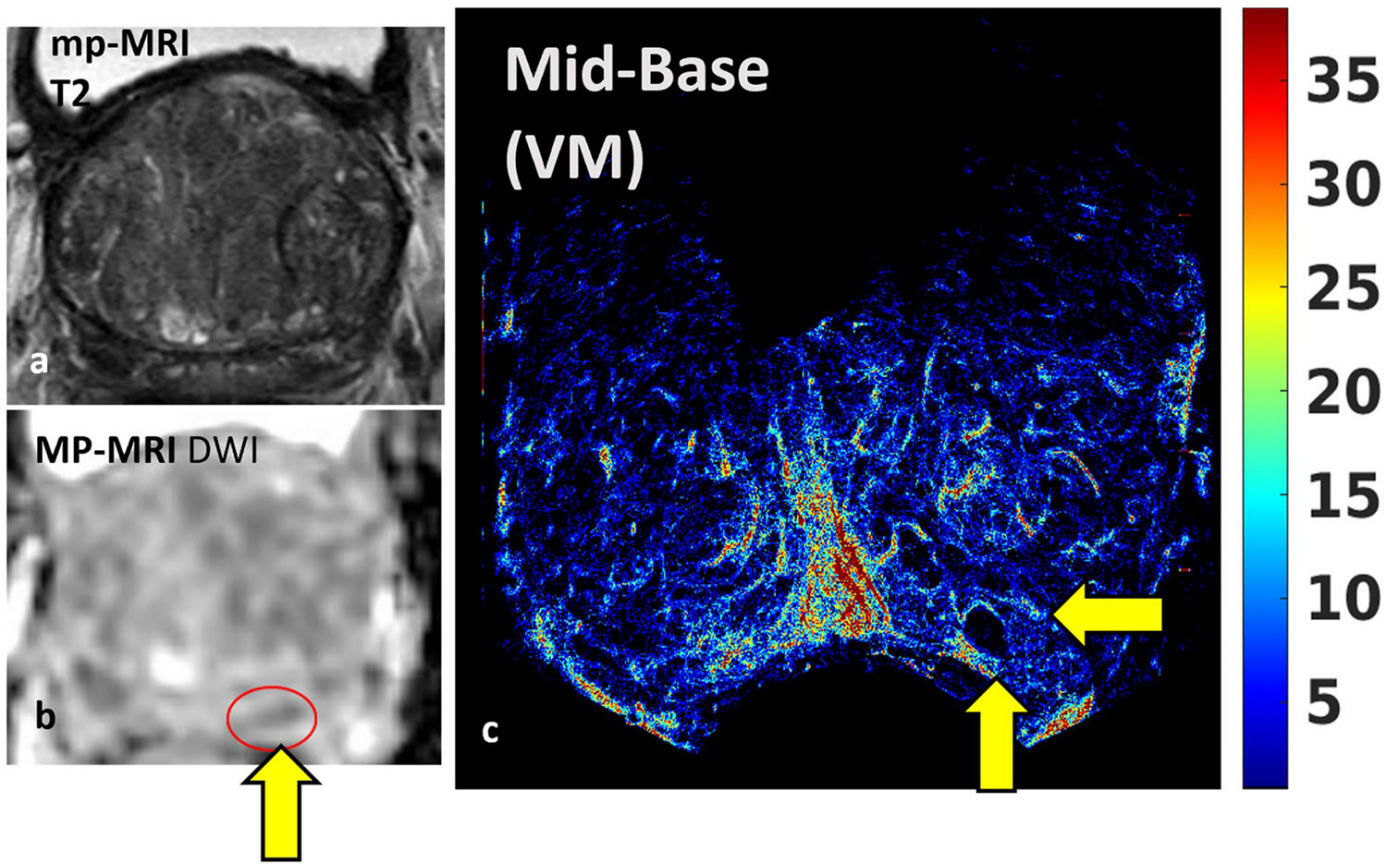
Multiparametric magnetic resonance imaging (mpMRI) compared with blood flow super-resolution ultrasound map for a prostate where the cancer was found by biopsy in the left posterior area. a T2-weighted image for the mid-base slice in Participant 134 (T2cNxMx PI-RADS 4); b diffusion-weighted image where a yellow arrow shows the region of suspicion; c blood flow super-resolution ultrasound mid-base map by vascular model (VM). While mpMRI (and biopsy) found a suspicious lesion in the mid-prostate in the left peripheral zone (b), in the super-resolution blood flow map, we see an avascular region alongside a smaller region of increased flow on either side (yellow arrows). A table containing the number of tracks and links is provided in the supplemental material (Table S7). As with previous data sets, the VM model provides a map containing fewer false tracks, and more correct tracks, created from fewer and shorter links
